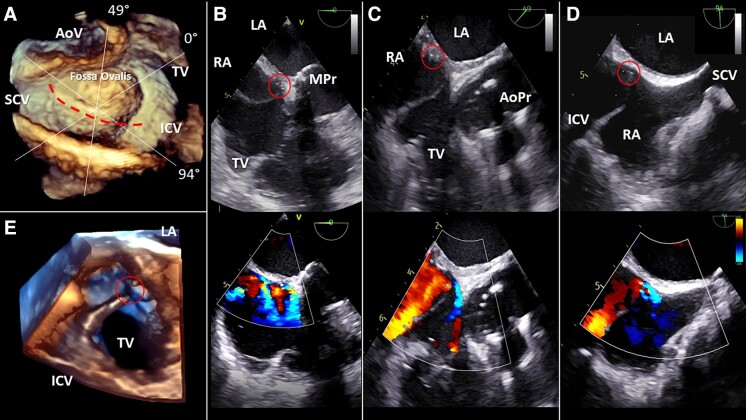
A 70-year-old woman underwent surgical replacement of aortic valve and mitral valve by bio-prostheses and annuloplasty of tricuspid valve due to rheumatic disease. A bi-atrial trans-septal approach (*Panel A*, dotted red line) was used, and inter-atrial septal (IAS) incision was finally closed with a 4-0 prolene suture. Post-operative transthoracic echocardiogram (TTE) revealed a residual mild left-to-right shunt at the level of IAS. During the following years, the patient remained asymptomatic. About 7 years after cardiac surgery, TTE showed an increase of inter-atrial shunt. Transoesophageal echocardiography (TEE) revealed intimal layer debris on the right side of IAS (*Panel B*, upper; see Supplementary data online, *Videos S1* and *S2*), expanding into the right atrium (RA). A significantly prominent eustachian valve was involved by dehiscence at its insertion point on the IAS (*Panels C* and *D*, upper; see Supplementary data online, *Videos S3–S5*). Small hyperechoic points (red circles) denoted the residual of stitches. Colour Doppler mode revealed left-to-right shunt originating from multiple sites, probably along the suture line (*Panel B*, bottom; see Supplementary data online, *Video S6*), and exiting into the RA above the eustachian valve (*Panels C* and *D*, bottom; see Supplementary data online, *Videos S7* and *S8*). Whether the blood flowing from inferior cava vein towards IAS might have contributed to damage IAS at a ‘locus minoris resistentiae’ is unknown. This is the first report of IAS dehiscence as a consequence of incomplete closure of bi-atrial trans-septal incision. TEE is essential to formulate diagnosis, and volumetric rendering (*Panels A* and *E*; see Supplementary data online, *Video S9*) allows to better understand the spatial relationships between cardiac structures.
